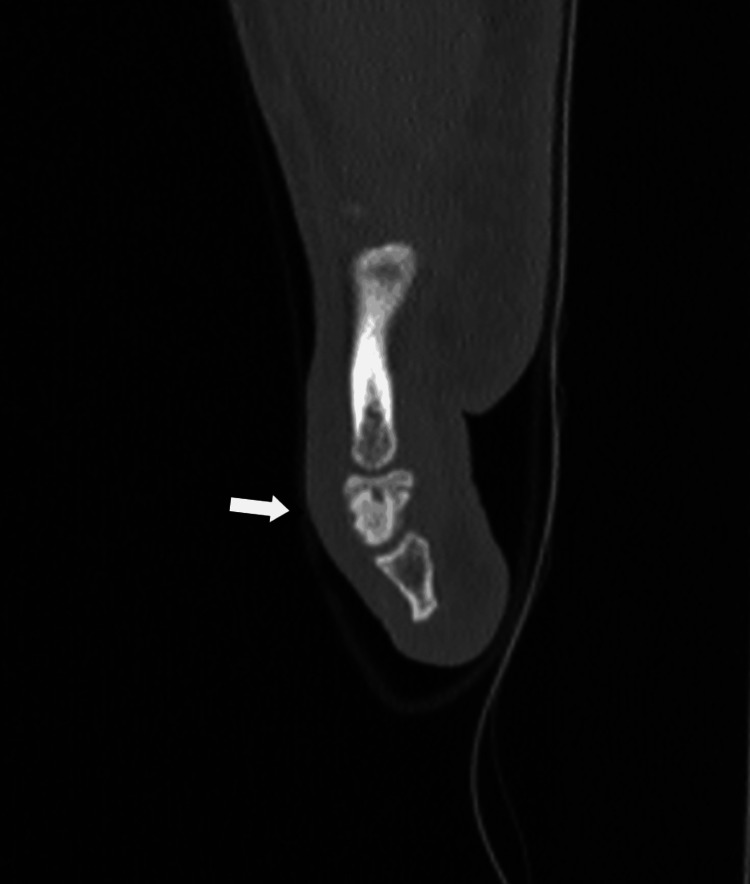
CT scan showing a centrally located sclerotic bone with a small radiolucent hypodense area in the transverse view
